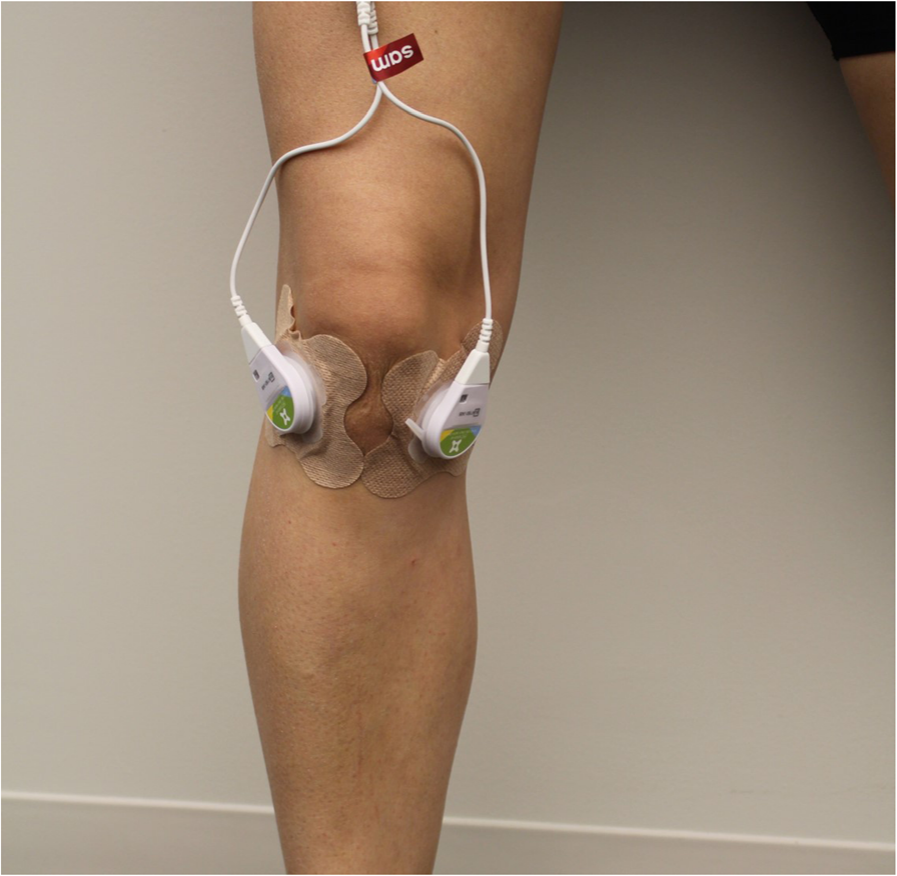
Wearable daily use ultrasound device. The wearable daily home-use long-duration low-intensity ultrasound device (SAM® Sport, ZetrOZ Systems LLC, Trumbull, CT) applied to the medial and lateral articulation points of the knee for the treatment of knee OA. The device is a prescription use only in the USA and has preconfigured ultrasound parameters of 3 MHz frequency and 1.3 W of energy for daily applied 4-h treatment
